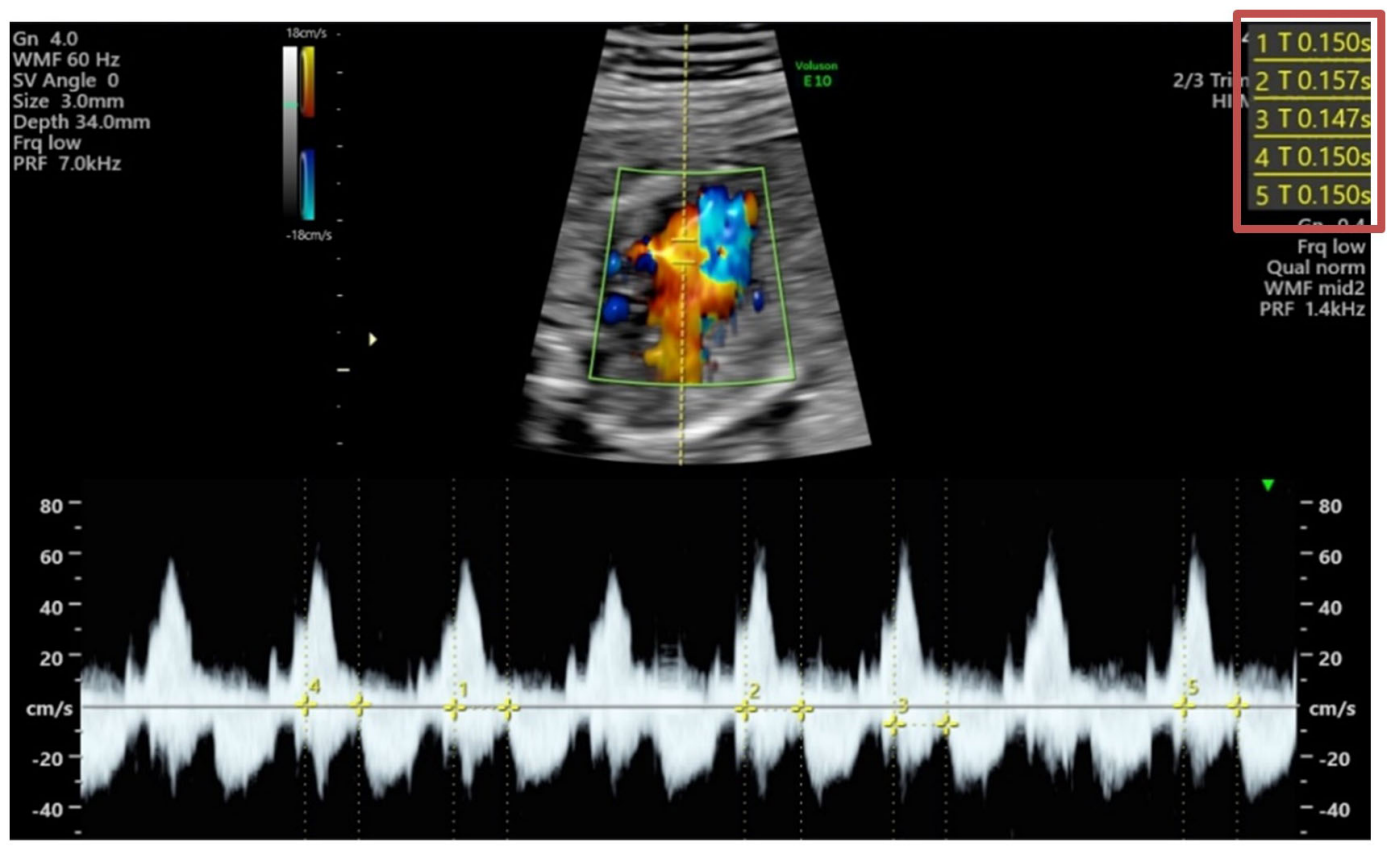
Fetal echocardiogram (2D image with color Doppler and flow Doppler) at 19 weeks of gestation, showing a prolongation of mechanical PR interval. On the top right corner of the image are presented the different measurements: 150, 157, 147, 150, 150 milliseconds.
